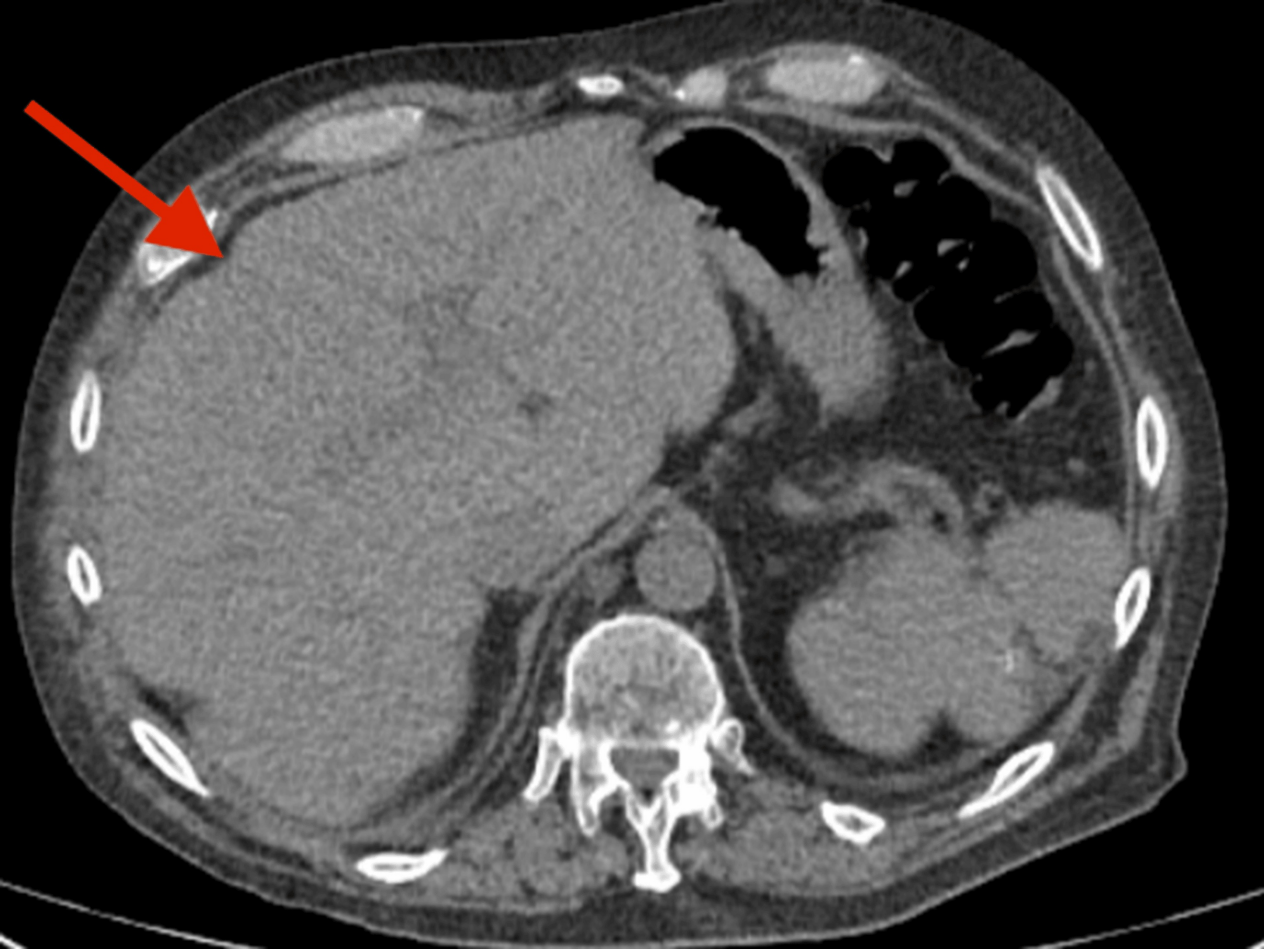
CT Abdomen Showing Cirrhotic Liver and Percutaneous Endoscopic Gastrostomy (PEG) Tube (26/02/2025) CT abdomen with contrast performed on 26 February 2025 showing a cirrhotic liver (red arrow) and an appropriately positioned PEG tube. No signs of active gastrointestinal bleeding were identified.
